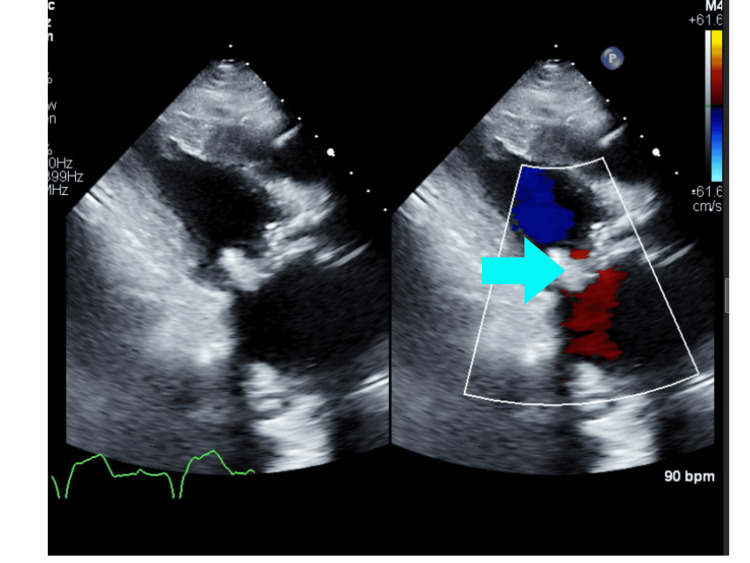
Echocardiography three months after surgery showing resolution of severe MS.
